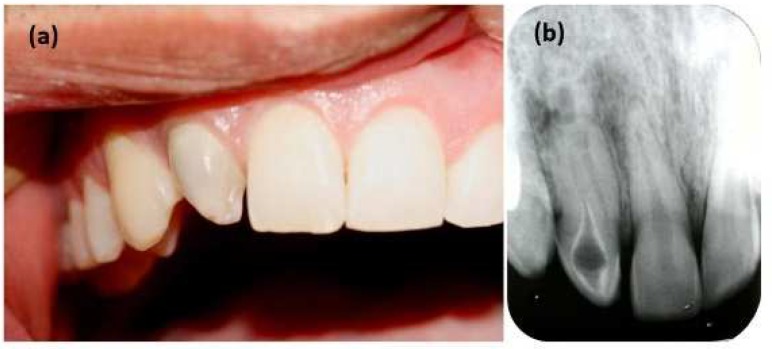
Preoperative; *A)* clinical image and; *B)* radiography of the maxillary right central incisor
